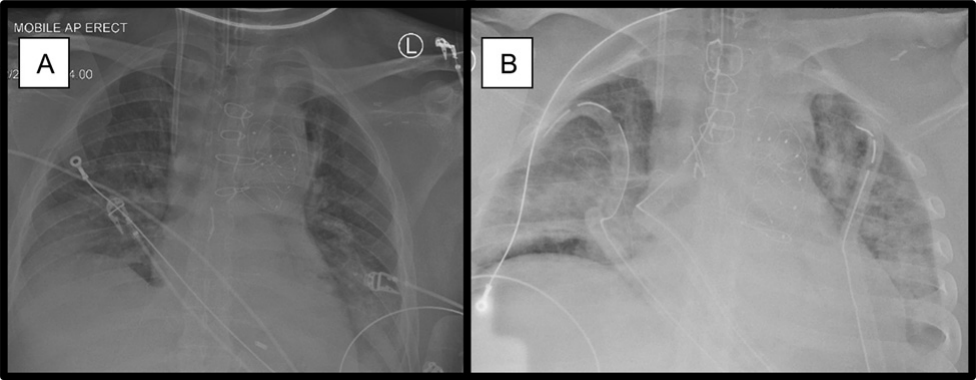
Anteroposterior erect chest X-rays: serial postoperative anteroposterior chest-X-rays taken on postoperative day 2 in the interval of 6 hours. Note the rapid progression of widespread bilateral ground-glass opacification, consisting bilateral pneumonitis.
